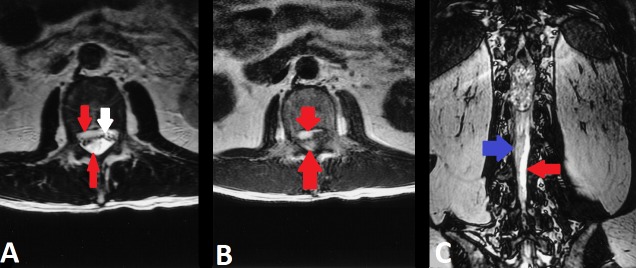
MRI spine Axial T2- [A] and T1-weighted [B] images show hyperintense subdural blood collections located both anterior and posterior (red arrows) to the cauda equina. The subdural blood is separate from the epidural fat signal intensity anteriorly [A, white arrow]. Coronal fast imaging with steady-state acquisition [C] shows the posterior blood collection (blue arrow) that is distinctly isolated from the hyperintense cerebrospinal fluid (red arrow)
